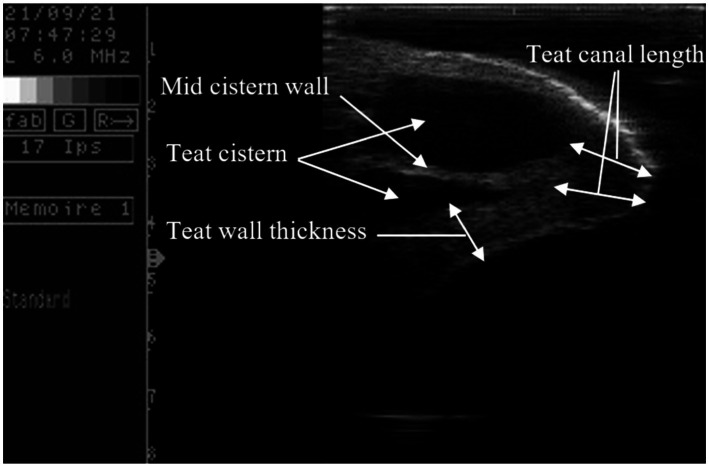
Measurements taken on camel teat ultrasound scan.
